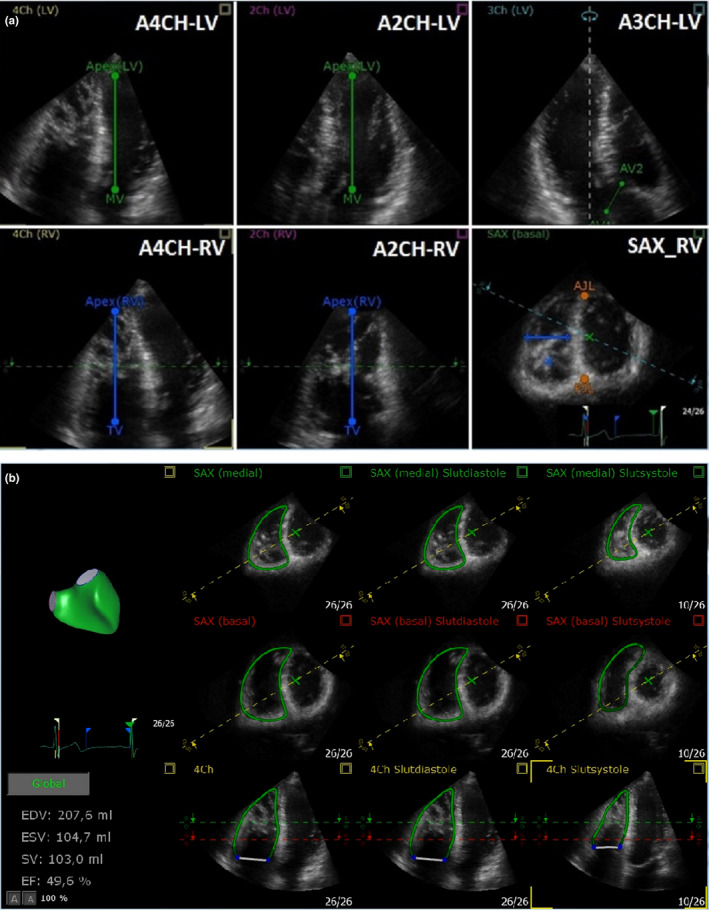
(a) Three‐dimensional echocardiographic analysis of the right ventricle. Three‐dimensional analysis of the right ventricle (RV), orientation and anatomic landmarks. In the first step, the user sets the left (top) and right (bottom) ventricular long axes in the apical four‐chamber view (A4CH) (A), apical two‐chamber view (A2CH) (B) at end‐diastole and the left ventricular outflow tract (LVOT) diameter is marked in the apical three chamber (A3CH) view (C, top). Than anterior (AJL, Anterior junction line) and posterior (PJL, posterior junction line) junctions of the right ventricular free wall with the interventricular septum, as well as the longest dimension of the right ventricular cavity between the septum and the free wall are set, both in a single short‐axis (SAX) view (C, bottom). AV aortic valve annulus; LV left ventricle; MV mitral valve; PJL posterior junction line; AJL anterior junction; TV tricuspid valve. (b) Three‐dimensional echocardiographic analysis of RV volume with TOMTEC. The software algorithm analyses the data and adapts the 3D model based on input information from the position of the requested anatomical landmarks. EDV, end‐diastolic volume; ESV, end‐systolic volume; SV, slag volume; EF ejection fraction; the white line represents tricuspid valve, 4Ch, four‐chamber view, SAX, short‐axis view
